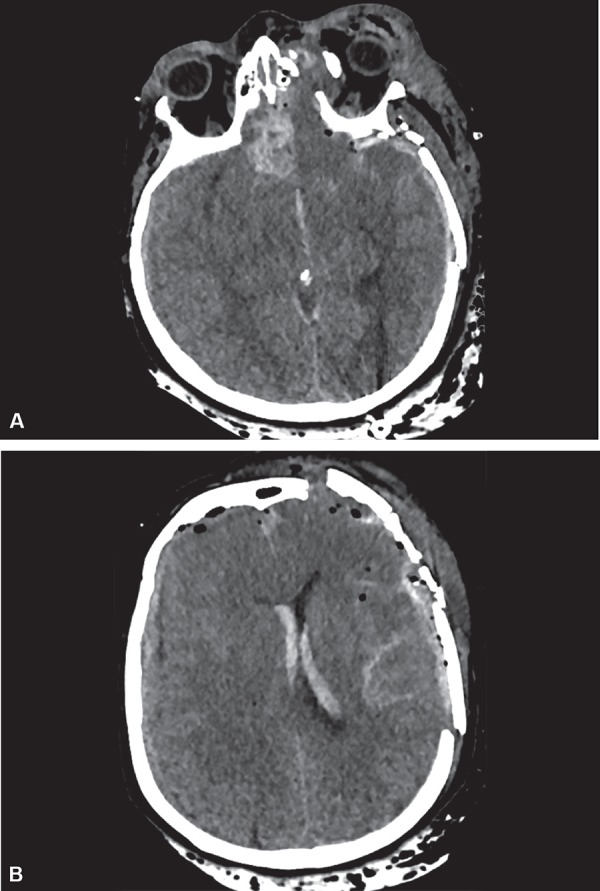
(A) Axial head CT scanner without contrast showing the right frontal lobe contusion; (B) Axial head CT scanner without contrast showing bilateral subdural hemorrhage, intraventricular hemorrhage, diffuse subarachnoid hemorrhage, pneumocephalus associated with depression of the frontal and parietal bones with fracture
